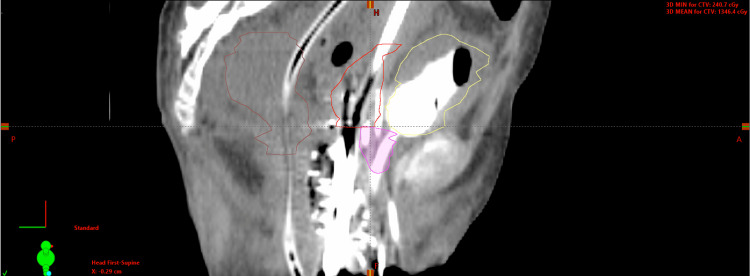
Sagittal CT showing HR-CTV (red) and CTV vagina (purple) The bladder and the rectum were contoured in yellow and brown, respectively.
